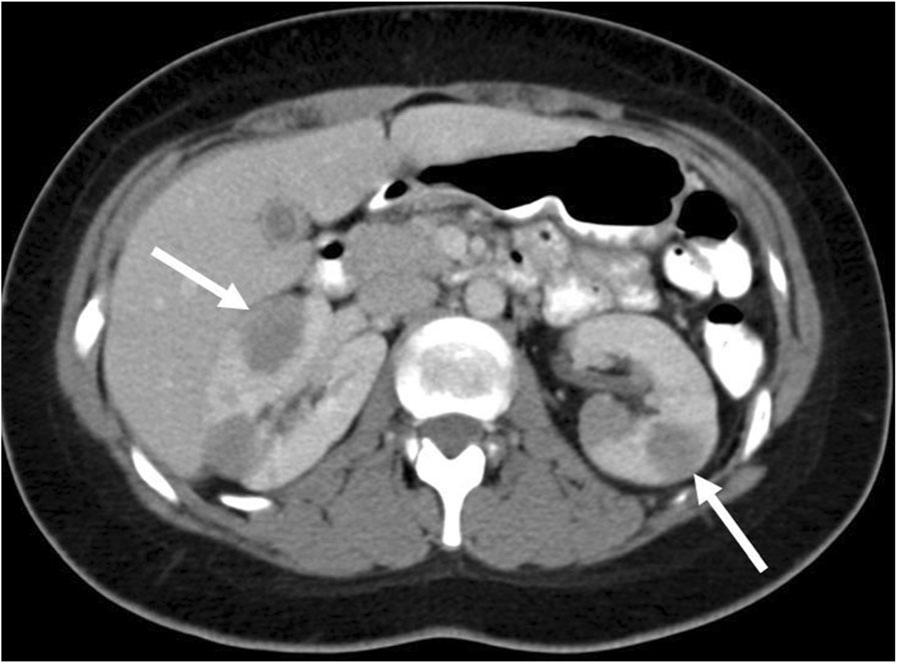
Renal involvement with renal masses. Contrast-enhanced axial CT image of a 23-year-old female with known Burkitt lymphoma demonstrates multiple circumscribed hypoattenuating renal masses (arrows)
